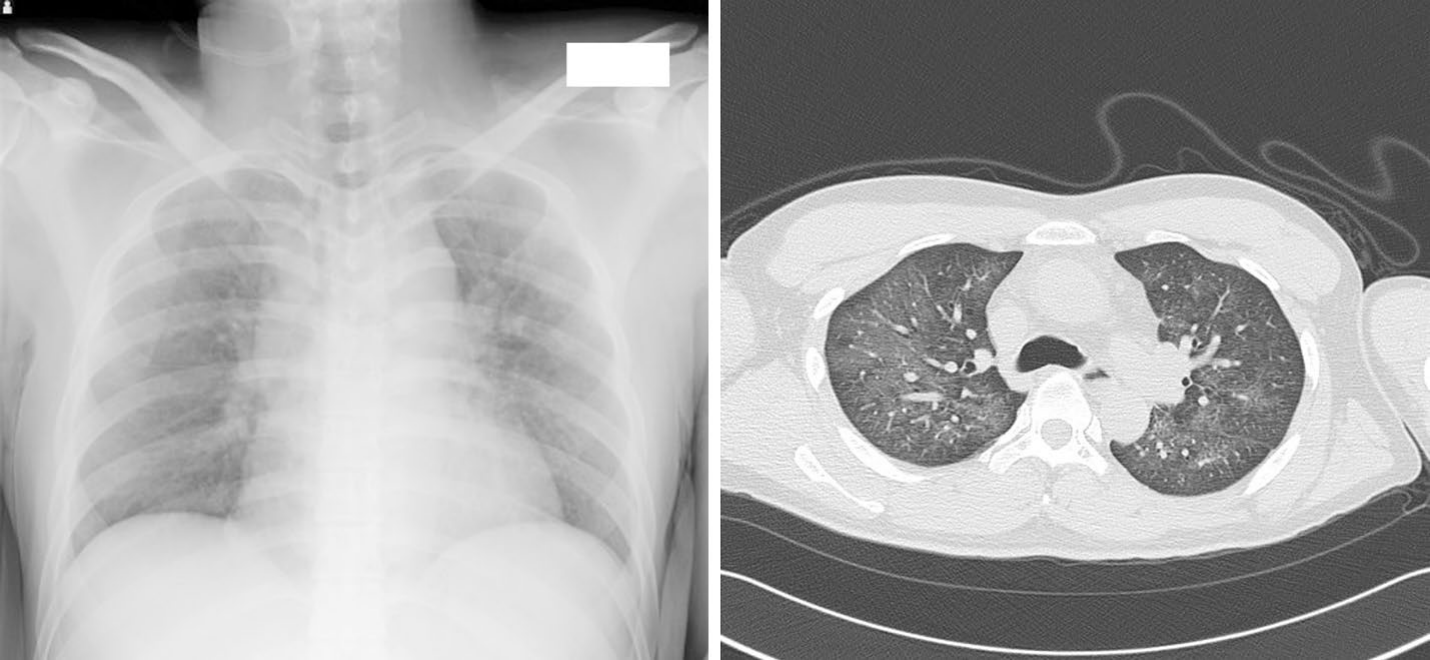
A radiograph (*left*) and high resolution CT scan (*right*) of the chest showing bilateral ground glass opacities.
